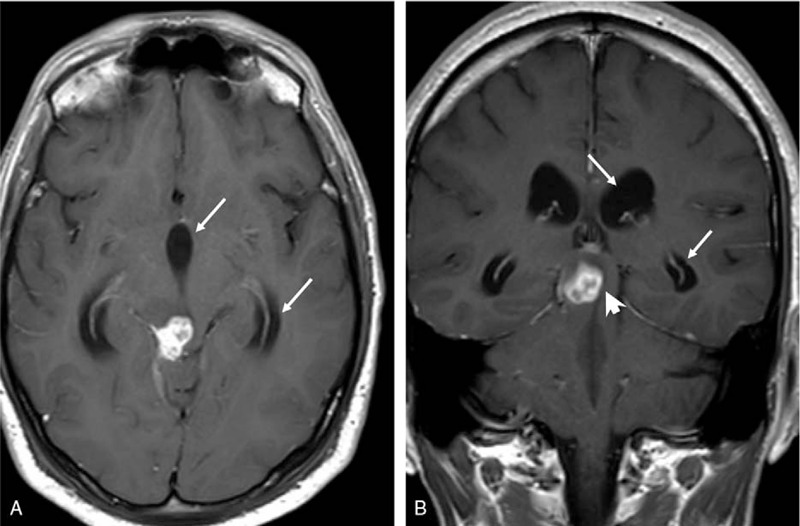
Detailed axial (A) and coronal (B) contrast-enhanced T1-weighted magnetic resonance images showing enlargement of III ventricle and temporal lateral ventricle horns (arrows) due to obstructive hydrocephalus secondary to mass effect over Silvius aqueduct (arrowhead).
